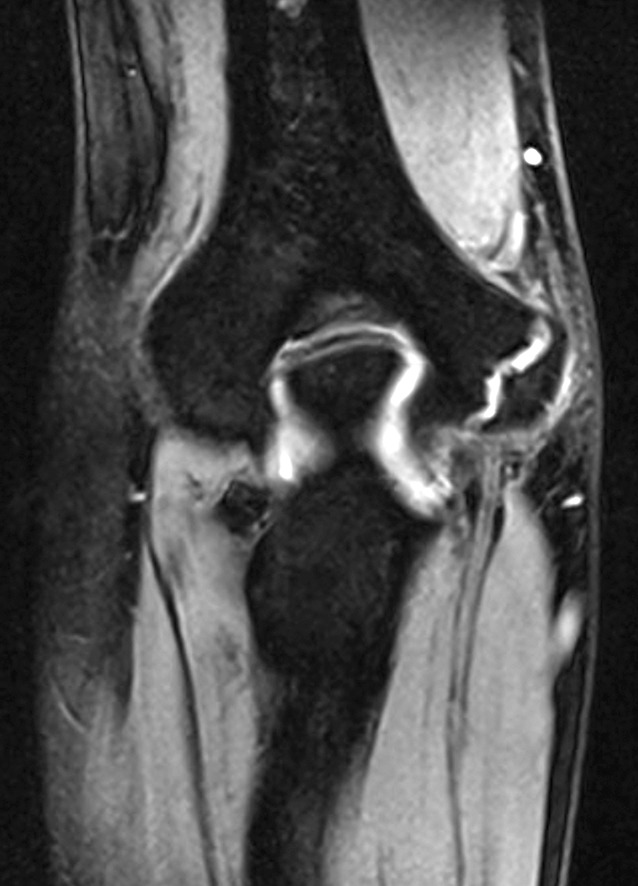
Magnetic resonance image of a medial epicondyle apophysitis in a skeletally immature athlete. (Courtesy of the MoRe Foundation)
